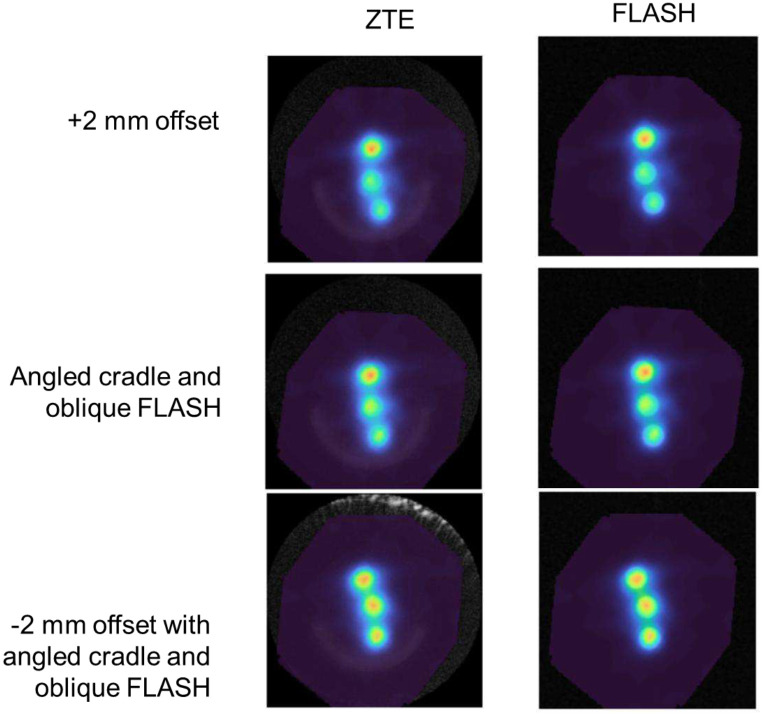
Registered PET images overlaid on the ZTE (left) and FLASH (right) MR images.
